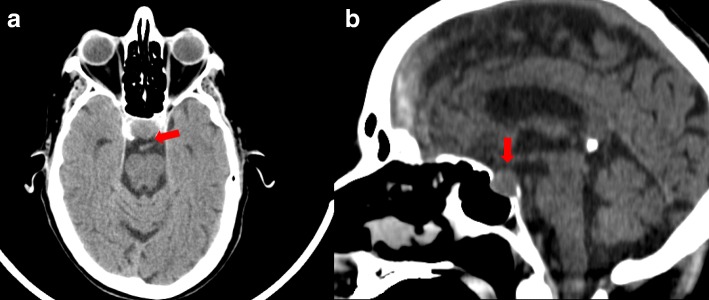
Arrows are pointing to the pituitary gland a and b Cranial computed tomographic scans showing an enlarged pituitary gland (15 × 20 × 14 mm) without calcification
